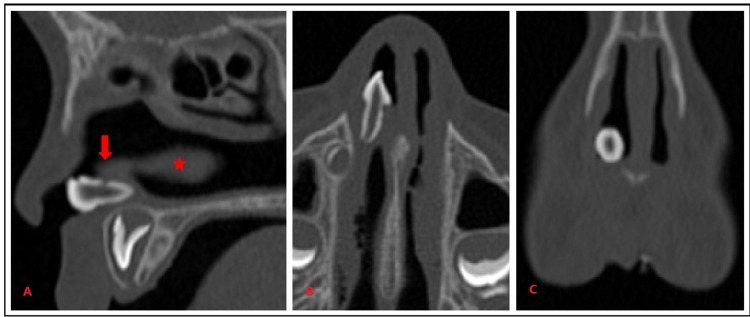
CT scan with bone window setting shows a homogeneous high attenuation lesion adjacent to inferior turbinate (red star), and surrounded by soft tissue at its base corresponding to the granulation tissue (red arrow) (A). The lesion showed a central slit-like radiolucency in axial view (B) and a focal central radiolucency in coronal view (C), both corresponding to pulp space.
